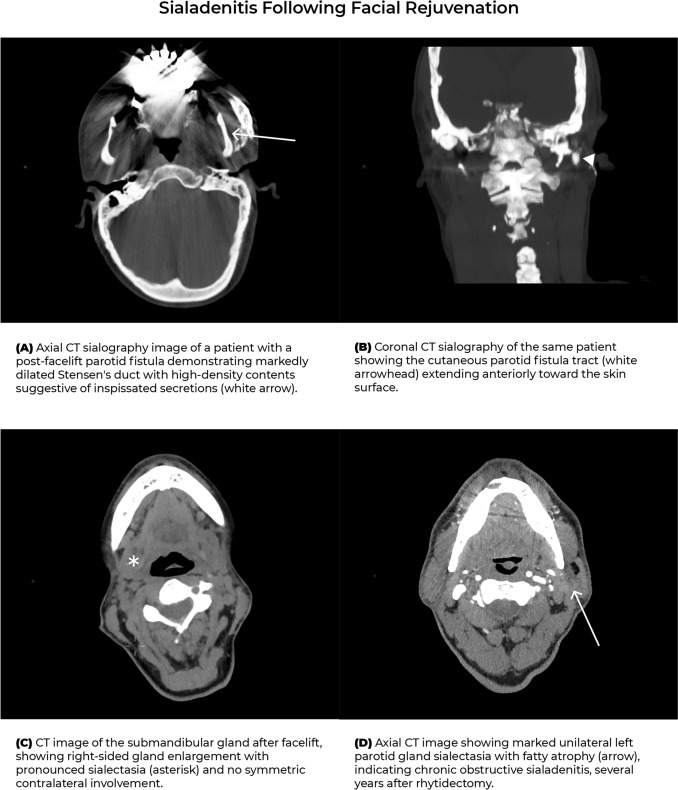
Representative radiologic and sialographic findings in patients with obstructive sialadenitis after facial rejuvenation procedures. A. Sialography showing markedly dilated left Stensen’s duct with turbid content and heavy sediment. B. Sialography showing contrast extravasation through a cutaneous fistula (arrow), confirming sialocutaneous tract formation. C. Axial CT image showing enlarged right submandibular gland with sialoectasia (arrowhead). D. Coronal CT confirming left-sided atrophy and asymmetric ductal disease (arrow)
